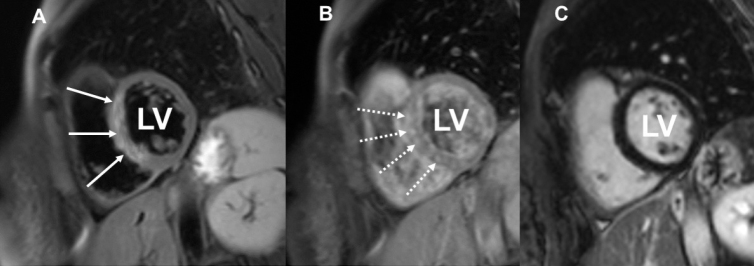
Cardiac MRI of a 38-year-old-woman with systemic sclerosis at baseline regional high T2 signal intensity at mid anteroseptal and inferoseptal segments of the left ventricle (A: white arrows), patchy mid wall non-ischemic pattern on early gadolinium enhancement sequence (B: dashed arrows), and no abnormal signal intensity of myocardium on late gadolinium enhancement (C). (LV: Left ventricle).
